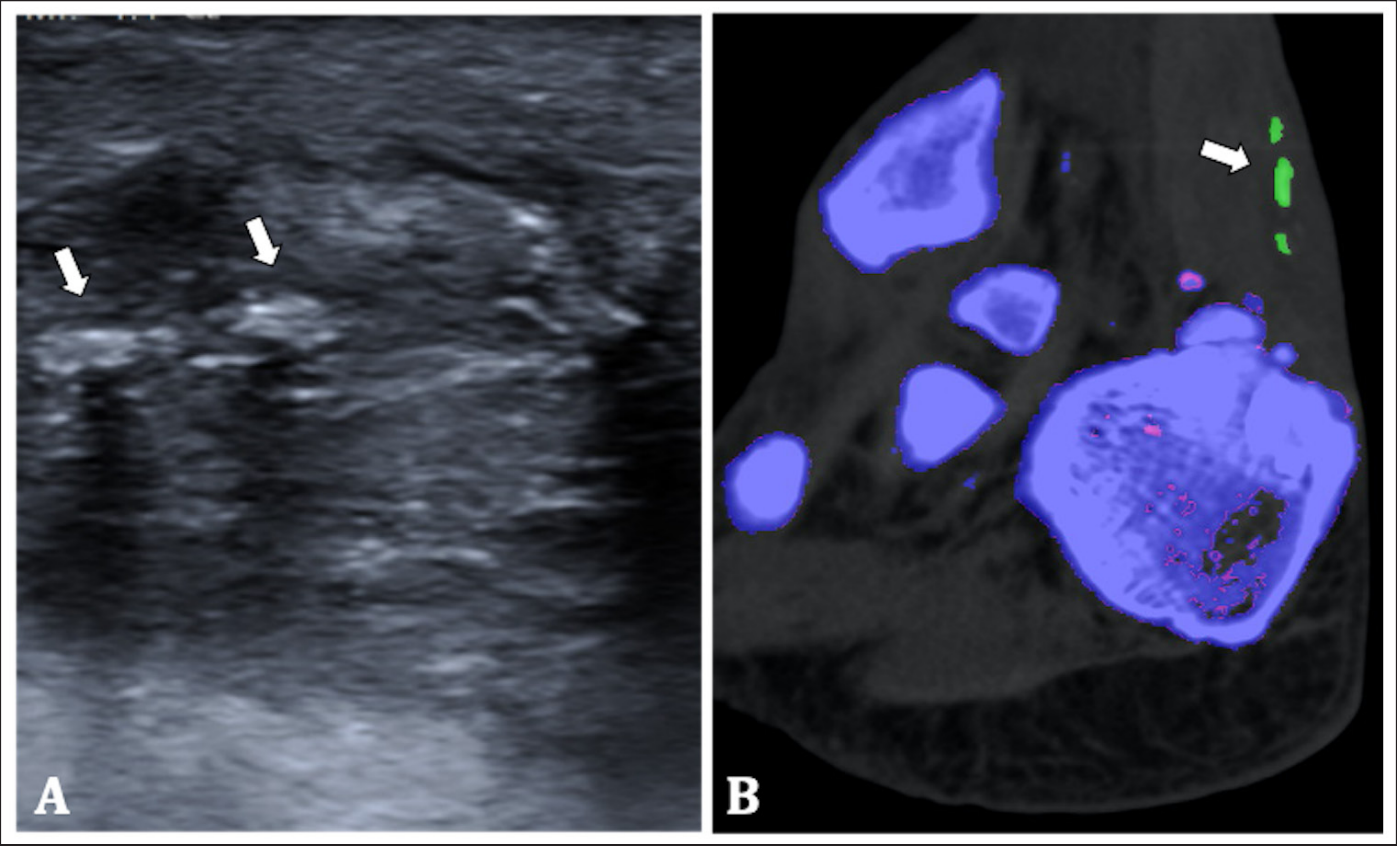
70-year-old man. (A) Ultrasound of the right Achilles tendon shows tendon thickening and multiple hyperechoic nodules (arrows). (B) Colour-coded DECT image shows MSU crystal deposition in the Achilles tendon (arrow).
